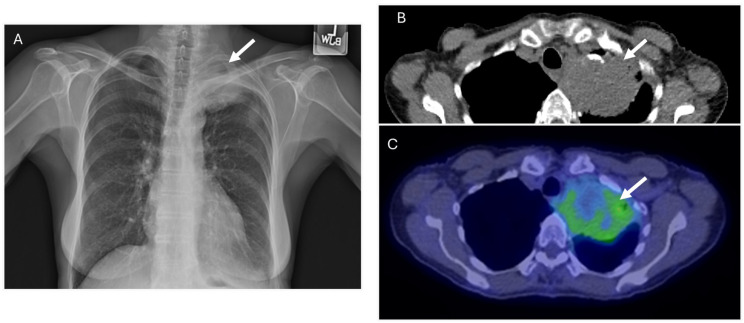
A 76-year-old female with left upper lung mass seen on radiograph ((A), arrow) taken after a bicycle accident. This was confirmed as a pulmonary mass on CT ((B), arrow) and with internal and peripheral FDG activity on FDG-PET imaging ((C), arrow). Percutaneous biopsy revealed pulmonary amyloidoma.
